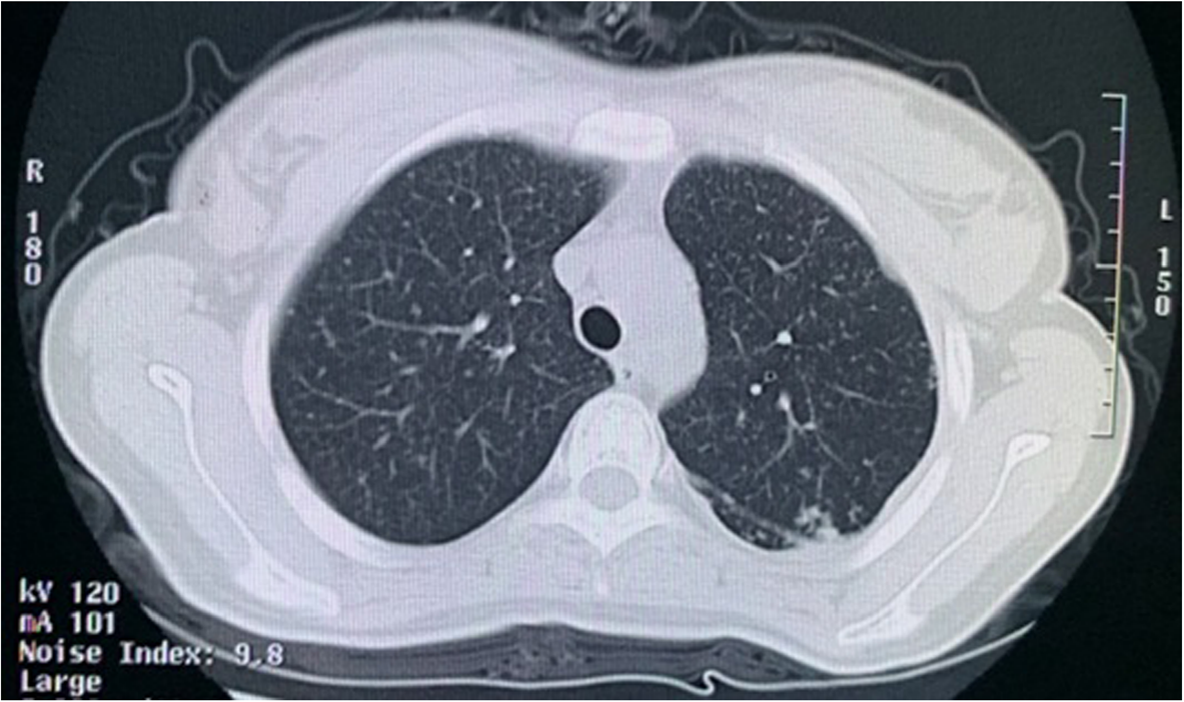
Representative chest computed tomography images showing multiple miliary nodules of uniform density, size and distribution
